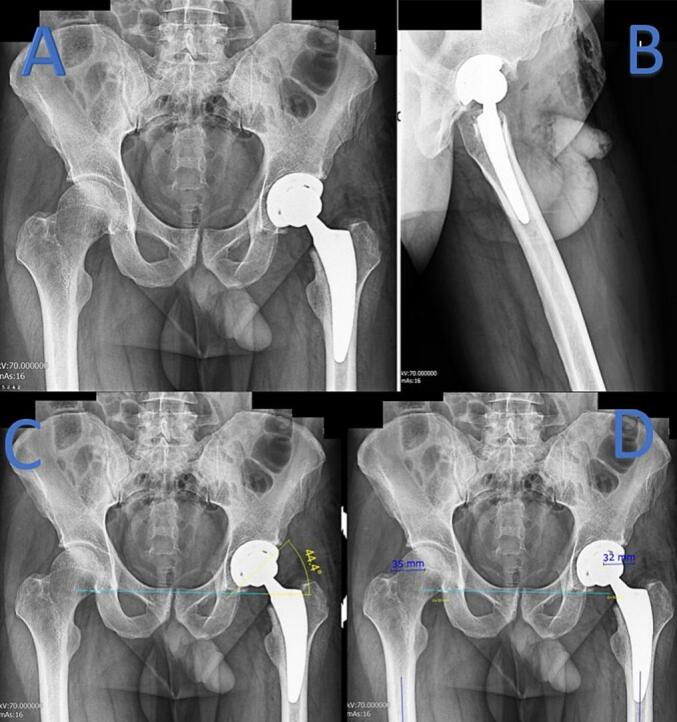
Post-operative pelvic x-ray. A: AP view, left hip successful prosthesis placement. B: Lateral view. C: Right (32 mm) and left (35) femoral offset. D: Left sharp angle (44.4°).
